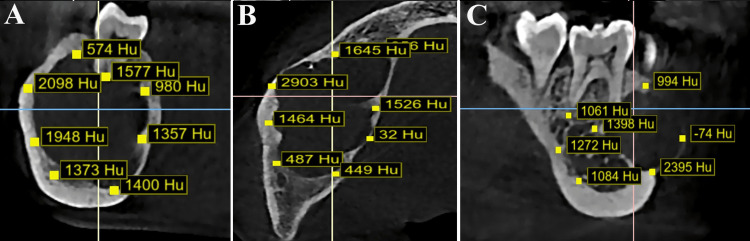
Cone-beam computed tomography images of mandible. Bone density (Hounsfield unit as Hu) measurements in nonaggressive mandibular radiolucent lesion: (A) oblique plane, (B) axial plane, and (C) sagittal plane Image credit: all images are original and related to case studies
